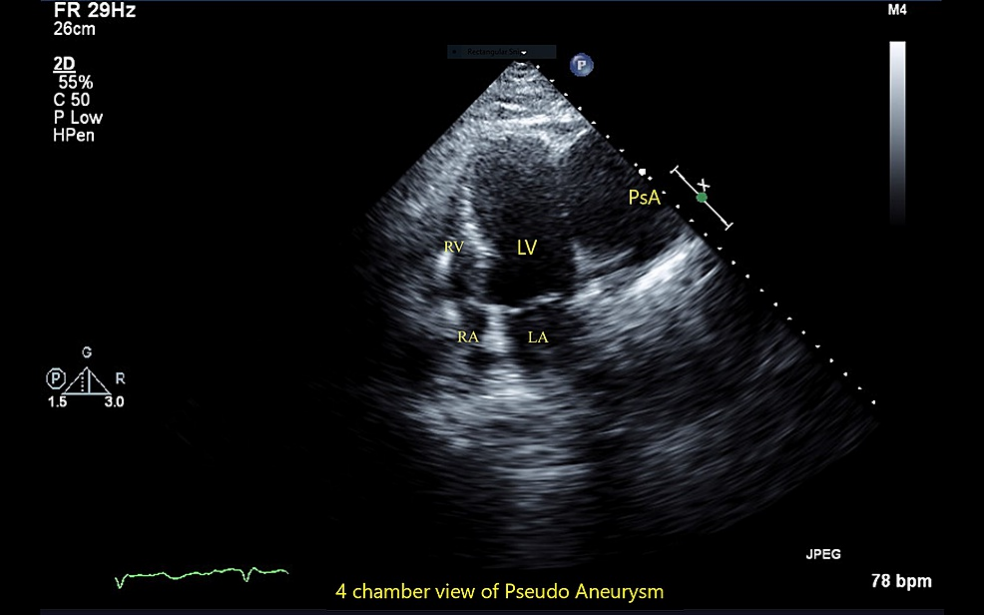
2D-TTE (apical four-chamber view) The image shows a thinned-out LV lateral wall and a contained LV free wall rupture with the formation of a large (8 cm) Pseudoaneurysm. 2D-TTE: Two-dimensional transthoracic echocardiography, LA: left atrium, LV: left ventricle, RA: right atrium, RV: right ventricle, PsA: pseudoaneurysm.
